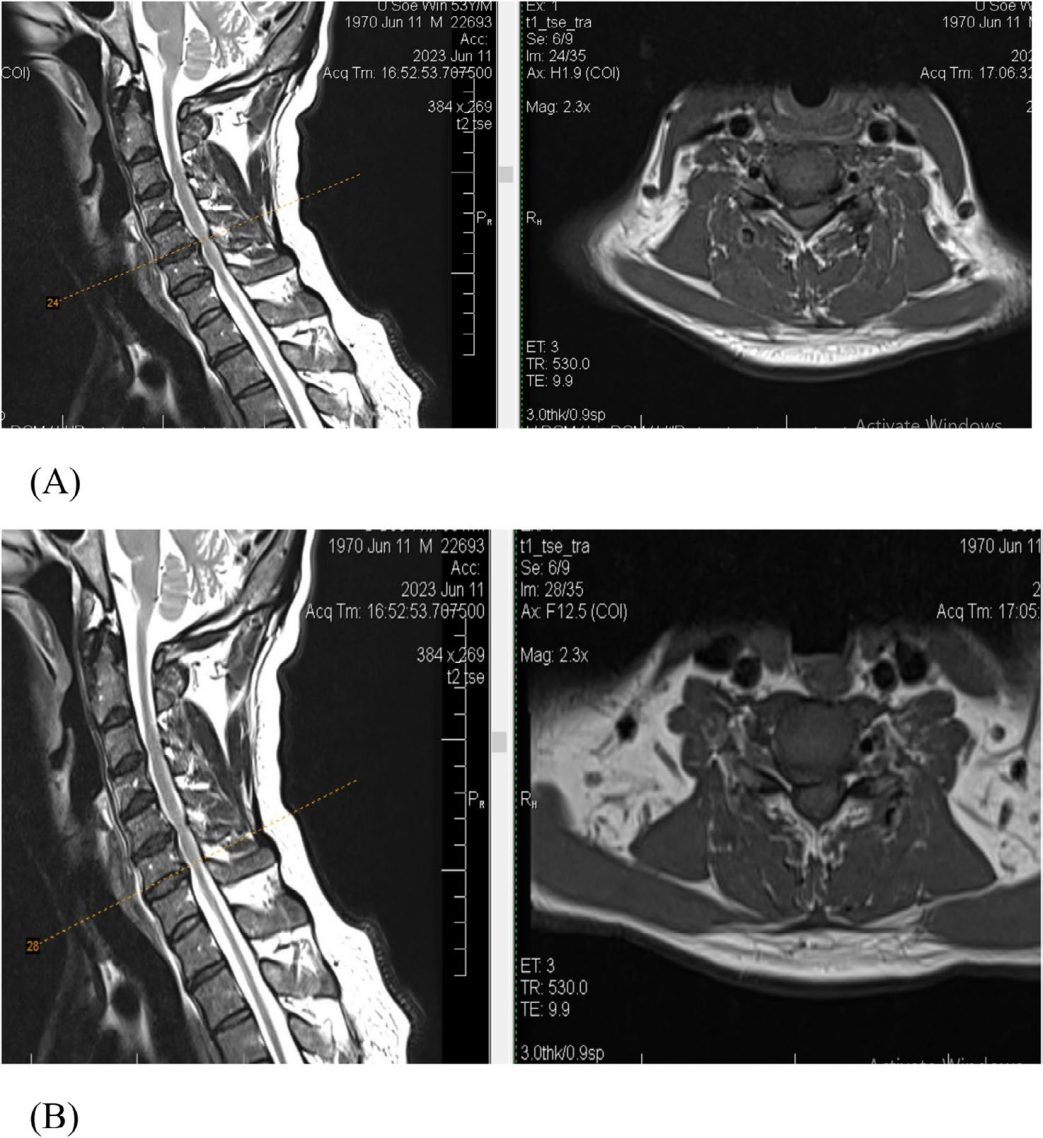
MRI images of the cervical spine demonstrating multilevel degenerative changes, most pronounced at the C5–C6 (A) and C6–C7 (B) levels. Notable findings include diffuse disc bulging, marginal osteophytes, ligamentum flavum thickening, causing spinal canal narrowing and moderate bilateral foraminal narrowing, and spinal cord T2 hyperintensity, consistent with cervical spondylotic radiculomyelopathy.
